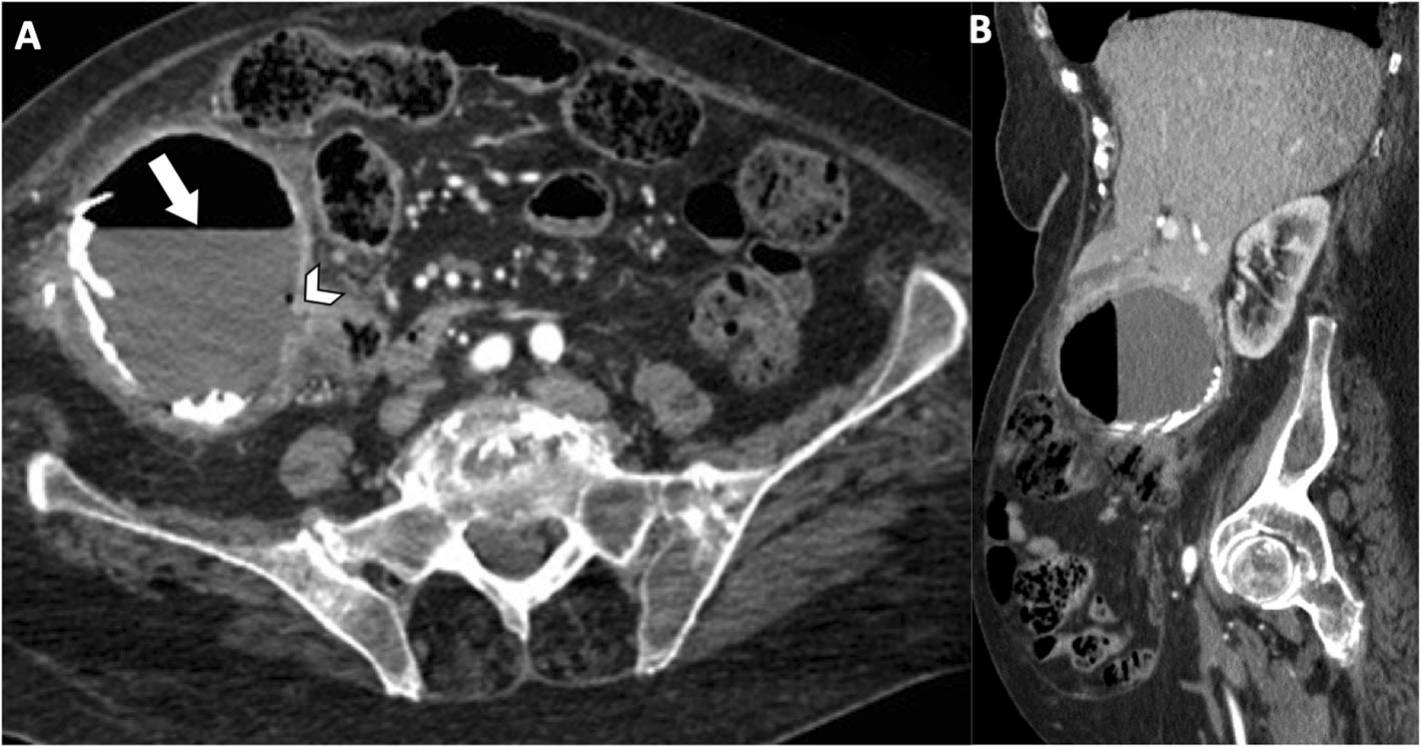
An 85-year-old woman with hydatid cysto-colic fistulization. Axial contrast-enhanced CT image (a) and sagittal reformatted CT image (b) on portal venous phase demonstrate a large hepatic hydatid cyst with peripheral wall calcifications and air-fluid level (arrow), with frank communication (arrowhead) with the right colon
